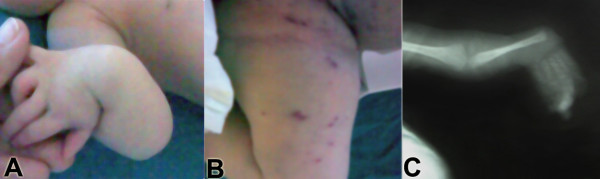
Clinical signs and X-ray findings in the baby girl. (A) Radial deviation of the right upper limb with no thumb deformity. (B) Multiple purpuric eruptions in the anterior aspect of the right lower limb. (C) X-ray of the left upper limb showing absent radius.
